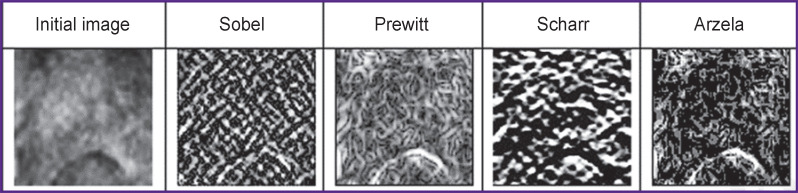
Various filters for isolation of contours on the image On images: MRI T1-WI axial with contrast enhancement, upper section of the Th6 thoracic vertebra
